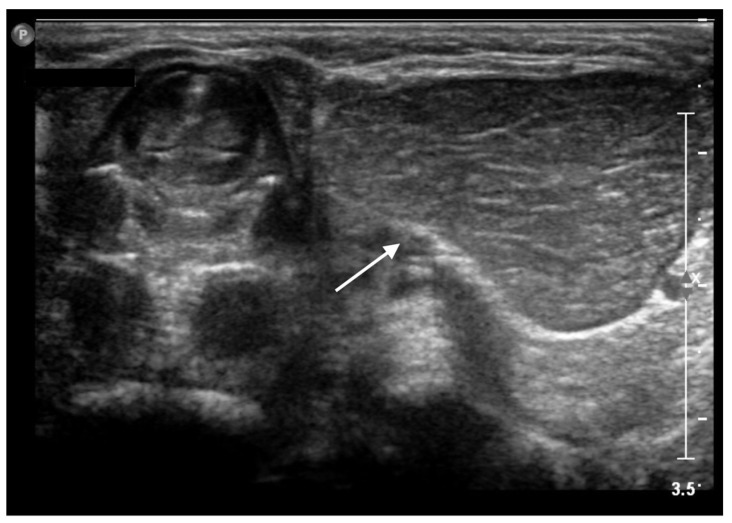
Transverse sonogram showing a 2 × 3 cm hypoechoic mass (*white arrow*) with linear hyperechoic foci, located to the left side of the cervical trachea.
